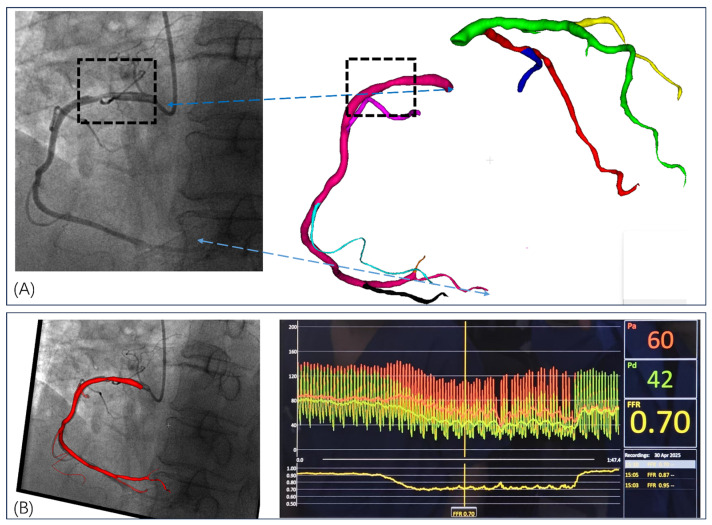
Invasive FFR Assessment of Right Coronary Artery Stenosis. (A) Coronary angiography showing significant stenosis in the proximal right coronary artery (dashed box) with corresponding 3D coronary tree reconstruction from 4D-CTA data. Color-coded segments represent different coronary branches used for computational analysis. (B) Real-time invasive FFR measurement displaying simultaneous aortic pressure (Pd: 60 mmHg) and distal coronary pressure (Pa: 42 mmHg) recordings during adenosine-induced hyperemia, yielding an FFR value of 0.70, indicating hemodynamically significant stenosis.
